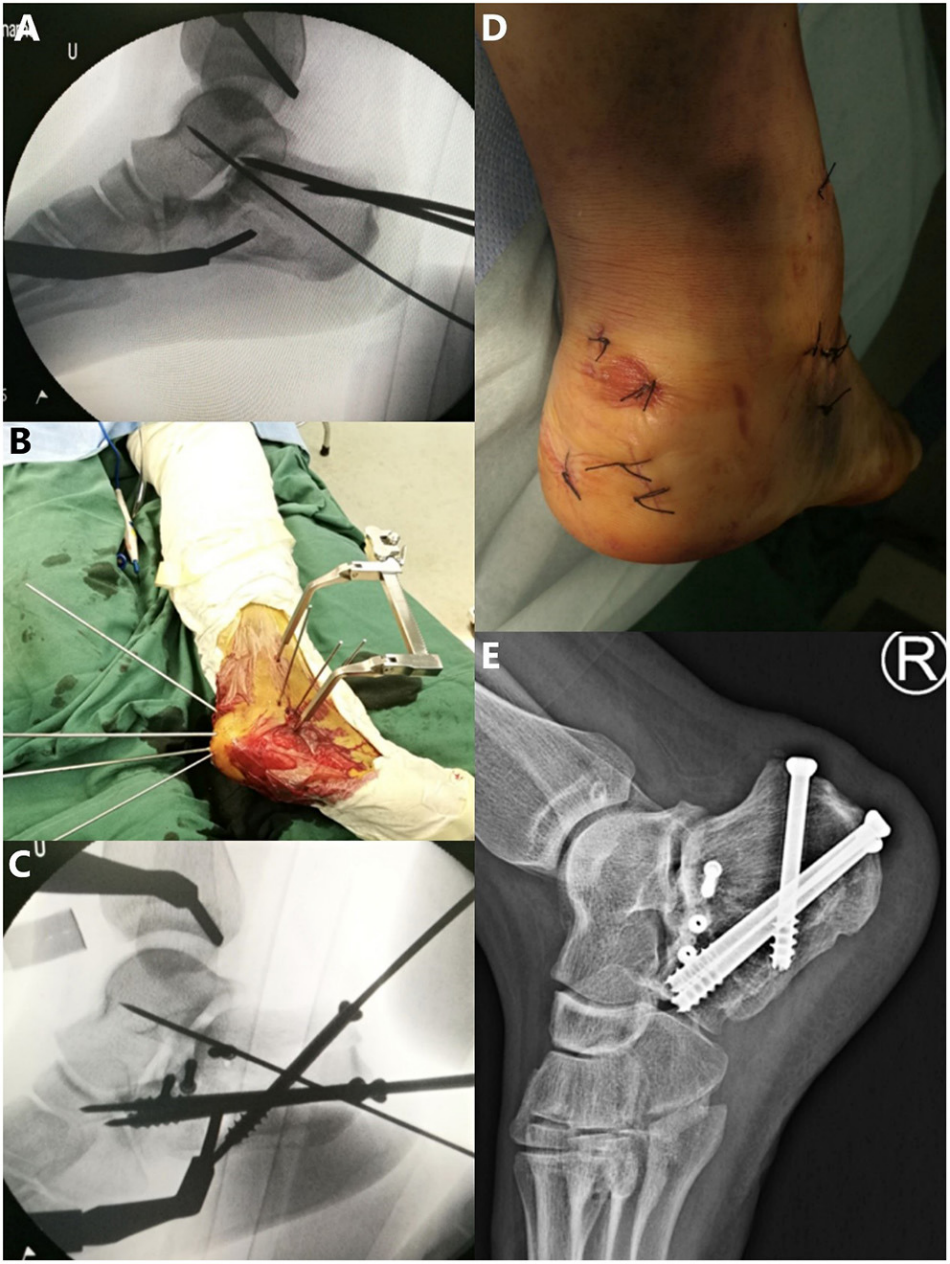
(A) In the operation on the foot in Figure 1, the Kirschner wire (2.0 mm) was inserted into the talus through the fracture line and the posterior face to maintain the reduction after the fracture was reduced by percutaneous leverage. (B,C) Guide pins were replaced percutaneously by appropriately sized cannulated screws. (D) Postoperative incision. (E) Lateral X-ray 12 months after the surgery.
